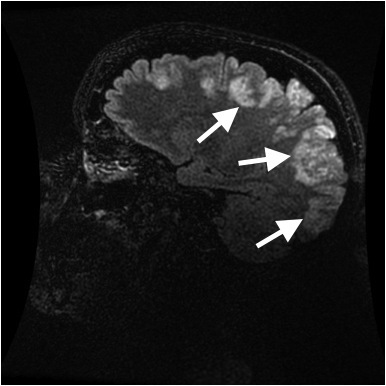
Initial T2 weighted magnetic resonance imaging of Patient 3 on second emergency department visit, showing increased T2 intensity throughout the cortical and subcortical gray matter of the bilateral frontal, parietal, occipital and posterior temporal lobes.
